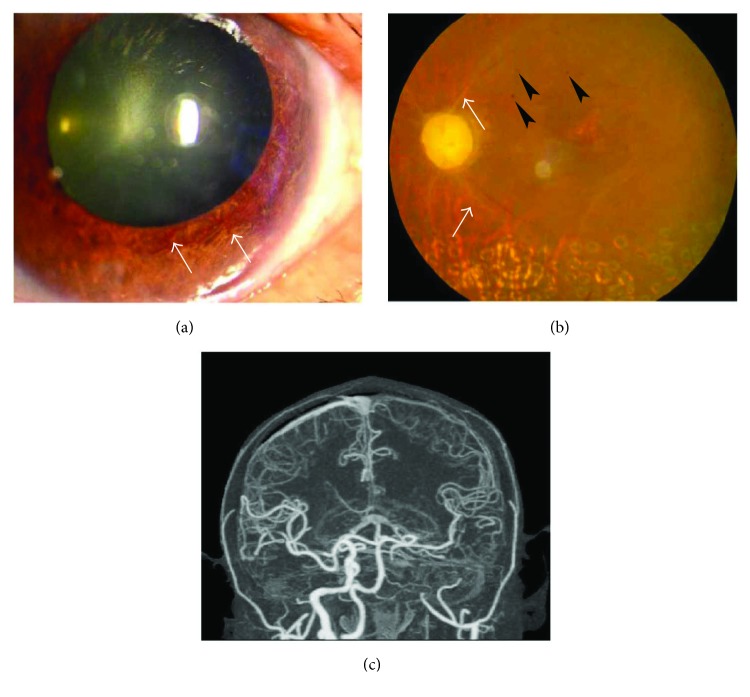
Slit-lamp photography (a), fundus photography (b), and computed tomographic angiography (CTA) (c) of a 60-year-old male OIS patient. (a) Rubeosis iridis (arrows) was found in the left eye. (b) The fundus of the left eye revealed a waxy optic disc, retinal arterial occlusion (arrows), and microaneurysms (arrowheads). This patient previously underwent retinal photocoagulation. (c) CTA demonstrated stenosis of the left ICA.
